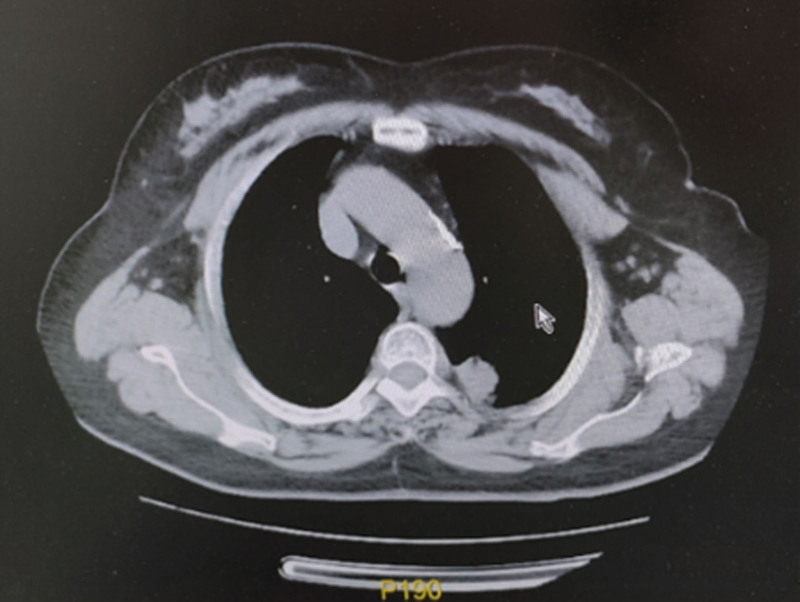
Mediastinal window. Plain chest CT (Figs. 1 and 2) revealed a clustered high-density shadow in the upper lobe of the left lung. CT = computed tomography.
